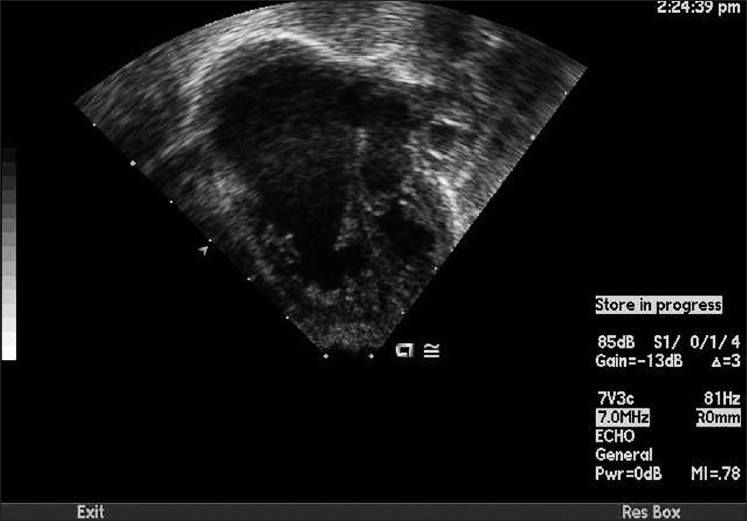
An apical four-chamber view demonstrating a massively dilated right atrium with distally displaced and dysplastic appearing tricuspid valve leaflets consistent with the Ebstein’s anomaly of the heart
